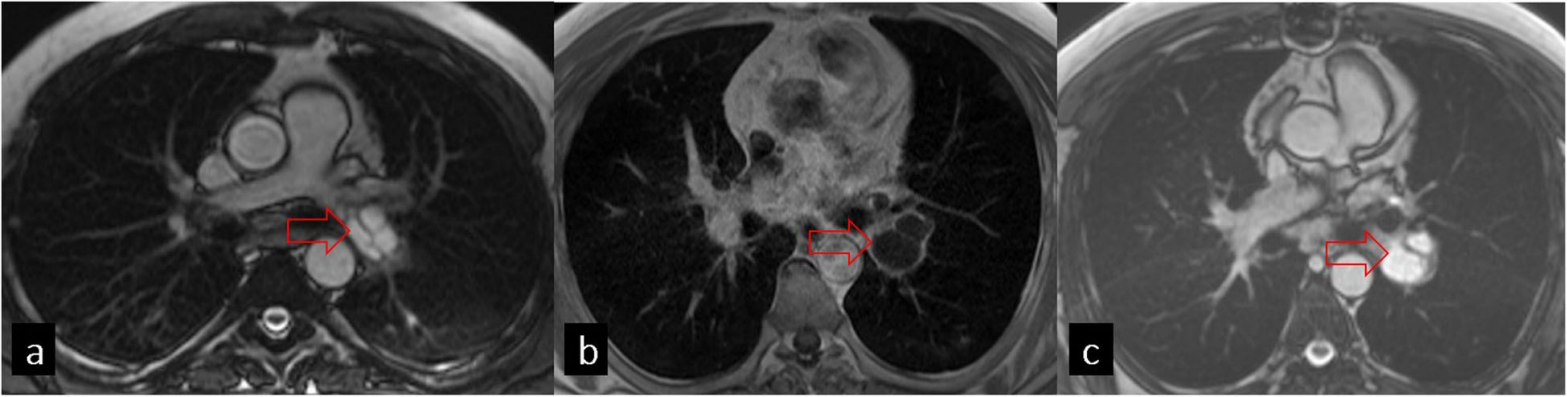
Steady-state free precession (SSFP) gradient echo magnetic resonance image shows a hyperintense hydatid cyst in the left pulmonary artery (a), contrast-enhanced T1W image shows hypointensity (b), and gradient TRUFI demonstrates hyperintensity in hydatid cysts (c)
